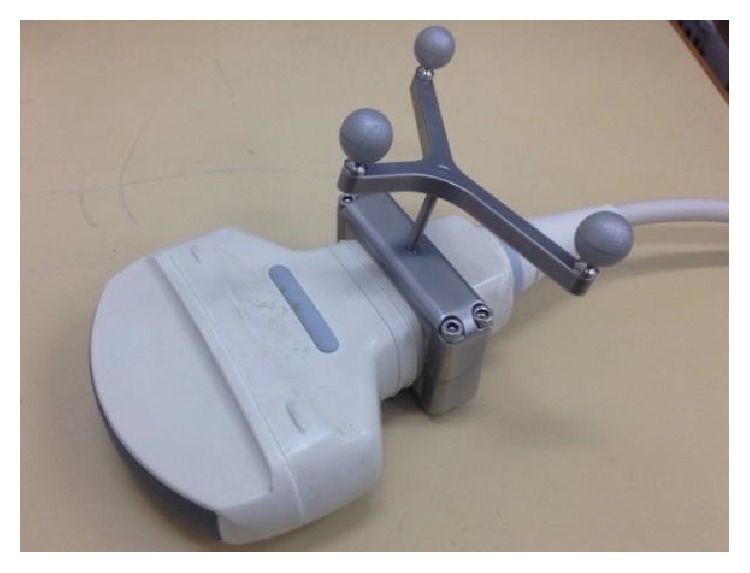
A DRF set on an ultrasound probe.
